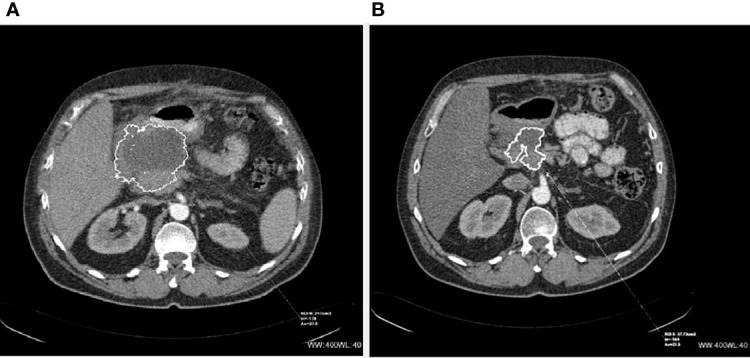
CT scans of inoperable (before and after PRRT) NET of the pancreas: (A) before PRRT and (B) 4 months after 4 cycles of 90Y-DOTA-TATE.
